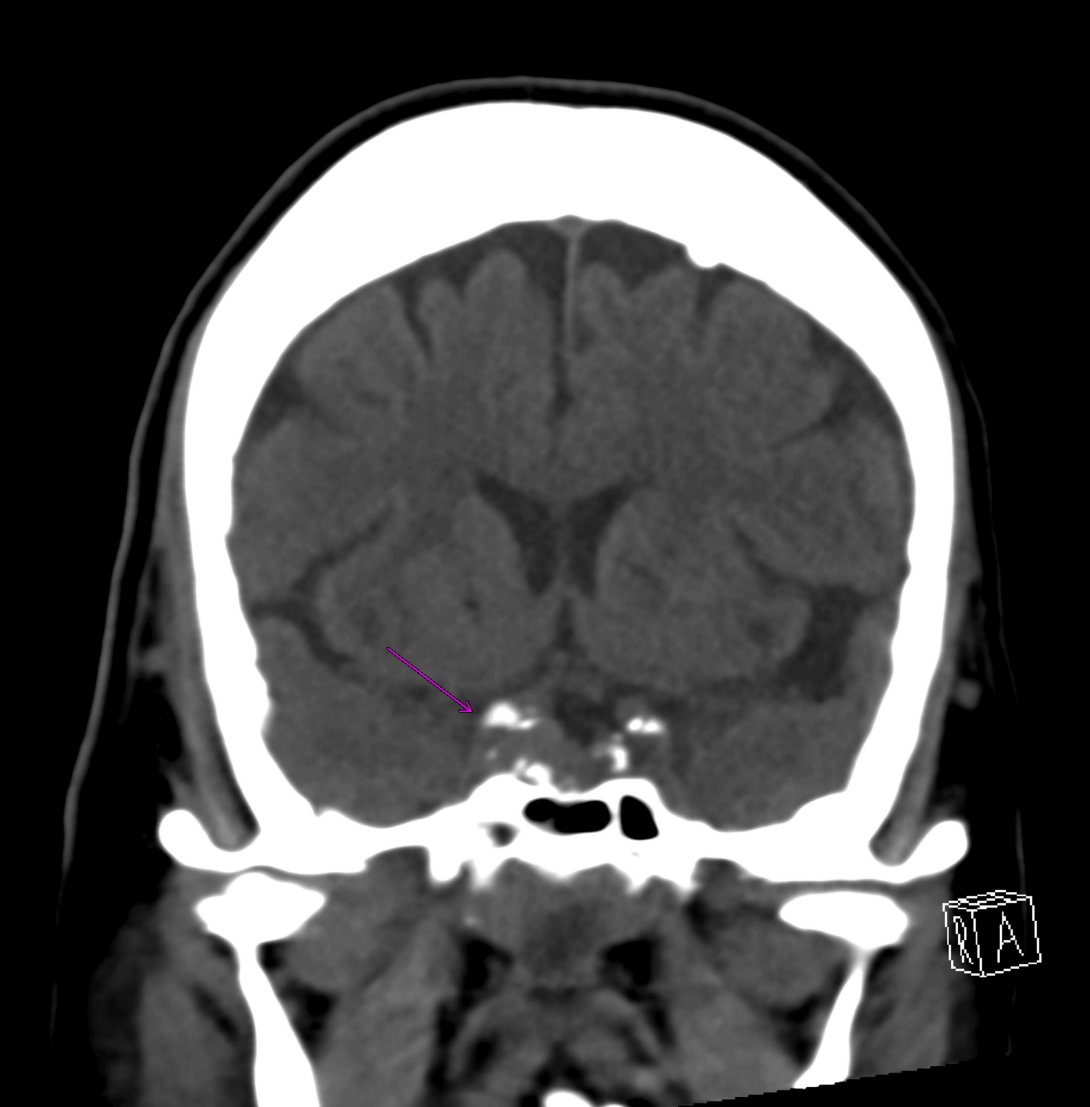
T1 fat-saturation post-contrast MRI demonstrating a T1 isointense, hypoenhancing lesion (pink arrow) in the right pituitary gland, which may represent a pituitary macroadenoma
